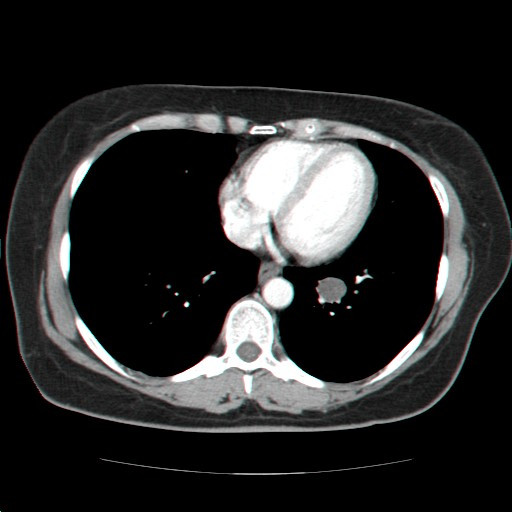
Axial contrast enhanced CT scan of the chest reveales the presence of a smooth edged 18 mm pulmonary lesion in the left inferior hilum.
